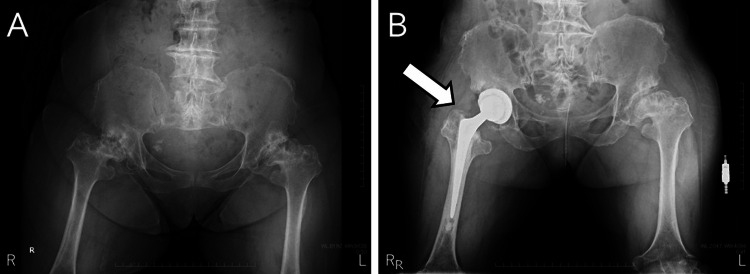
X-ray images of the hip: (A) preoperative and (B) postoperative.
